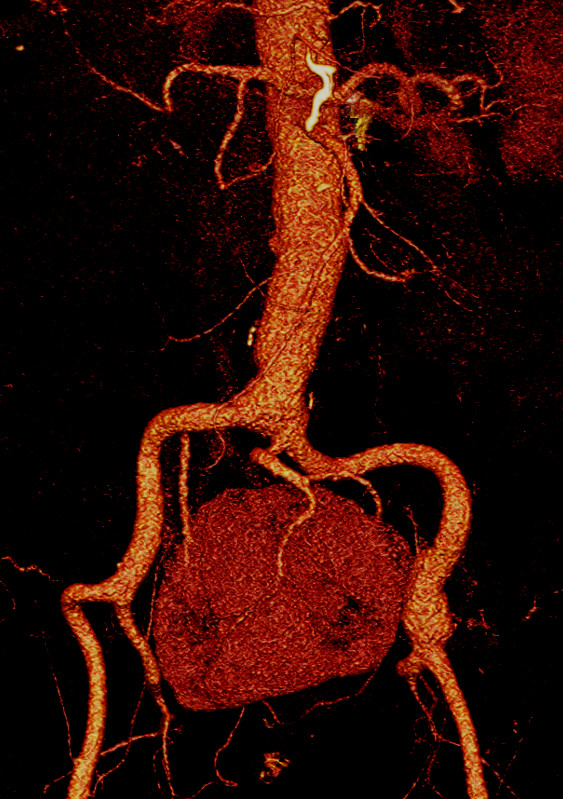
Follow-up two years after computed tomography angiography.
